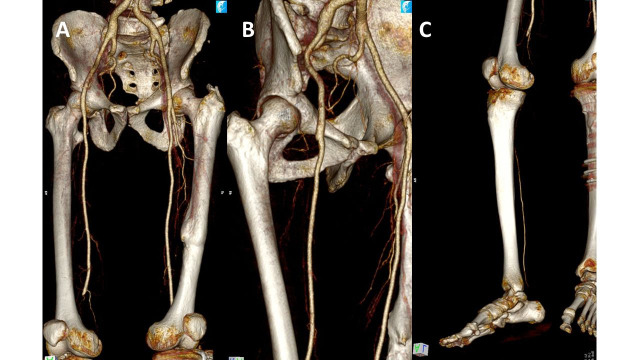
(A) Computed tomography angiography of the lower limbs showing occlusion of the popliteal segment; (B) deep femoral artery, and (C) the tibioperoneal trunk, with segmental refilling of the posterior tibial artery.
